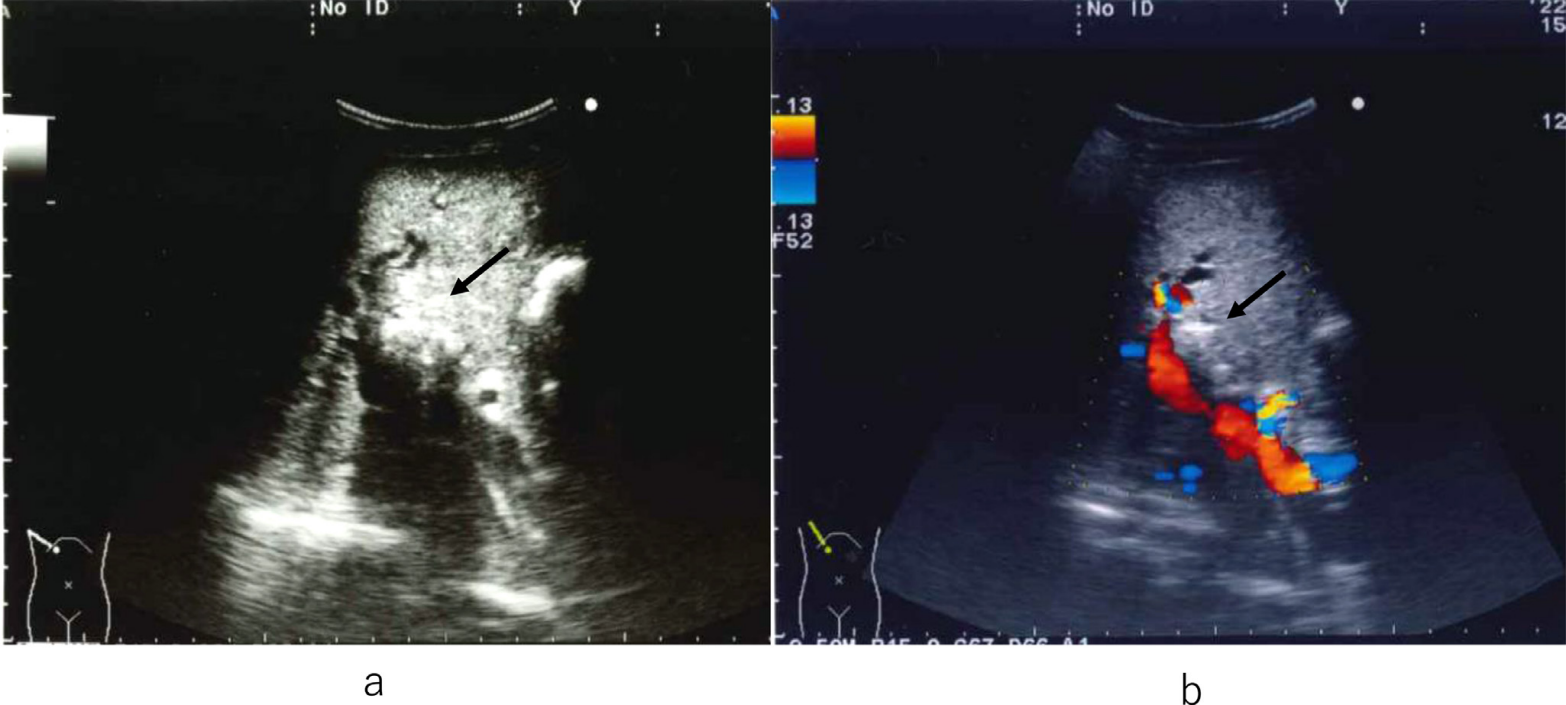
(A) Abdominal US shows high echoic lesions with acoustic shadow (arrow) (B) Color Doppler US before PTBD shows high echoic lesions (arrow) on the ventral side of the portal vein.
